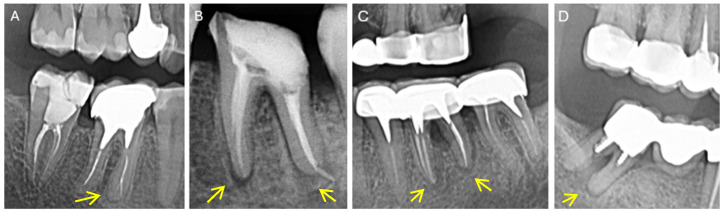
Endo-periodontal lesions (without root damage) in endodontically treated teeth with periapical lesions: (A) Grade 1 (4.6.–4.7) (B) Grade 2 (3.6) (C) Grade 3 (3.6.) (D) Grade 3 (4.7) (EPL classification according to Herrera et al., 2018 [26]).
